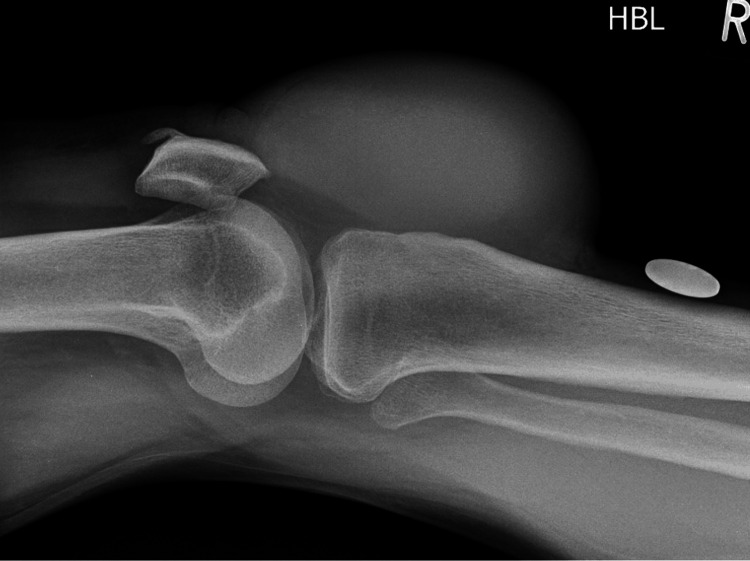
Right knee plain radiograph. HBL: horizontal beam lateral view; R: right
